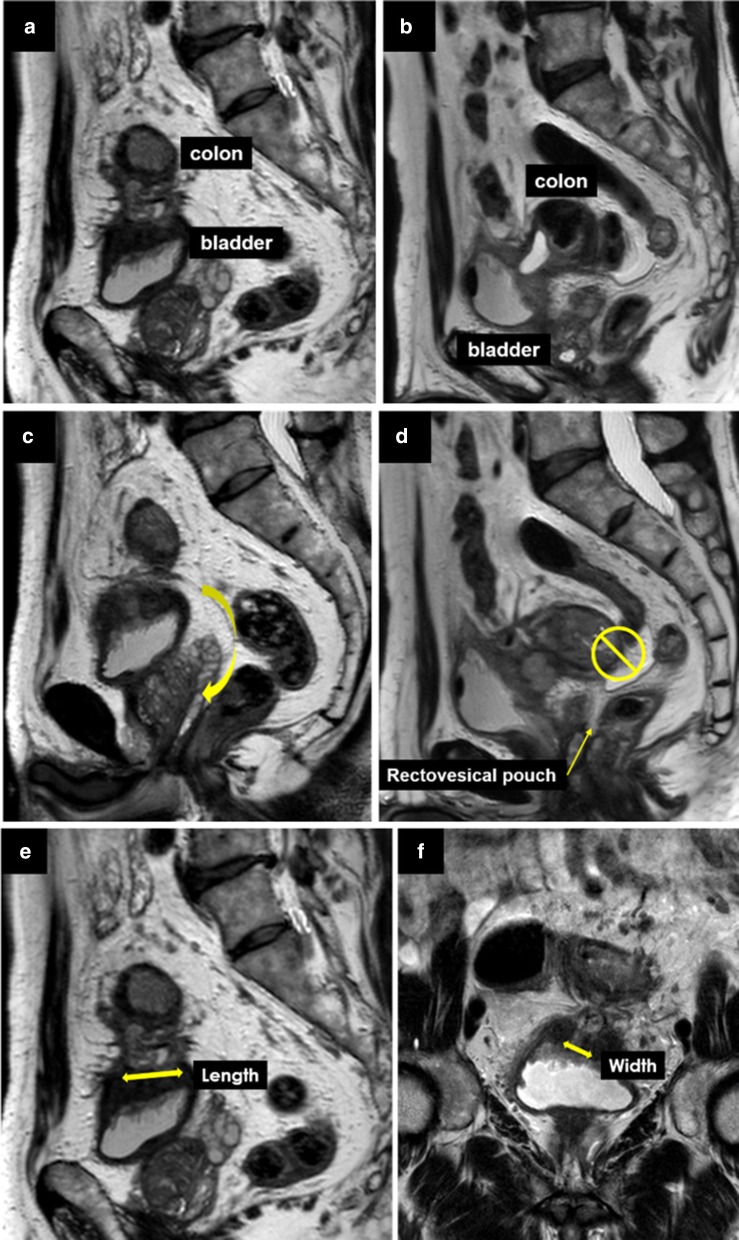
Fistula location on bladder and patency of the rectovesical pouch were evaluated using preoperative magnetic resonance images (MRI) from a representative case, and the estimated contact area between the sigmoid colon and bladder (eCA) was calculated as the product of the length and width between the sigmoid colon and bladder on two-dimensional MRI. a Fistula is located on the superior wall of the bladder. b Fistula is located in the posterior bladder. c: The patency of the rectovesical pouch is preserved and the fistula can be encircled. d The rectovesical pouch is closed and cannot be approached directly. e Length between the sigmoid colon and bladder on sagittal view. f Width between the sigmoid colon and bladder on coronal view
